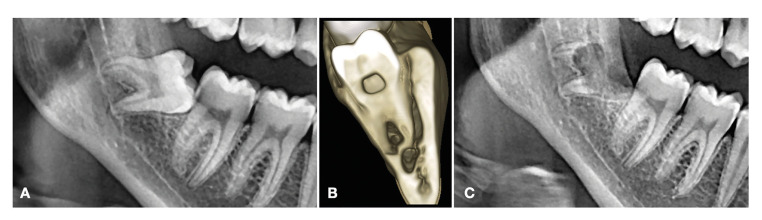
A) Right lower third molar appearing to be intimately related to the inferior alveolar nerve in the preoperative panoramic radiographic study. B) The inferior alveolar nerve crossed the two mesial roots of the right lower third molar. C) Postoperative panoramic radiographic view following coronectomy.
